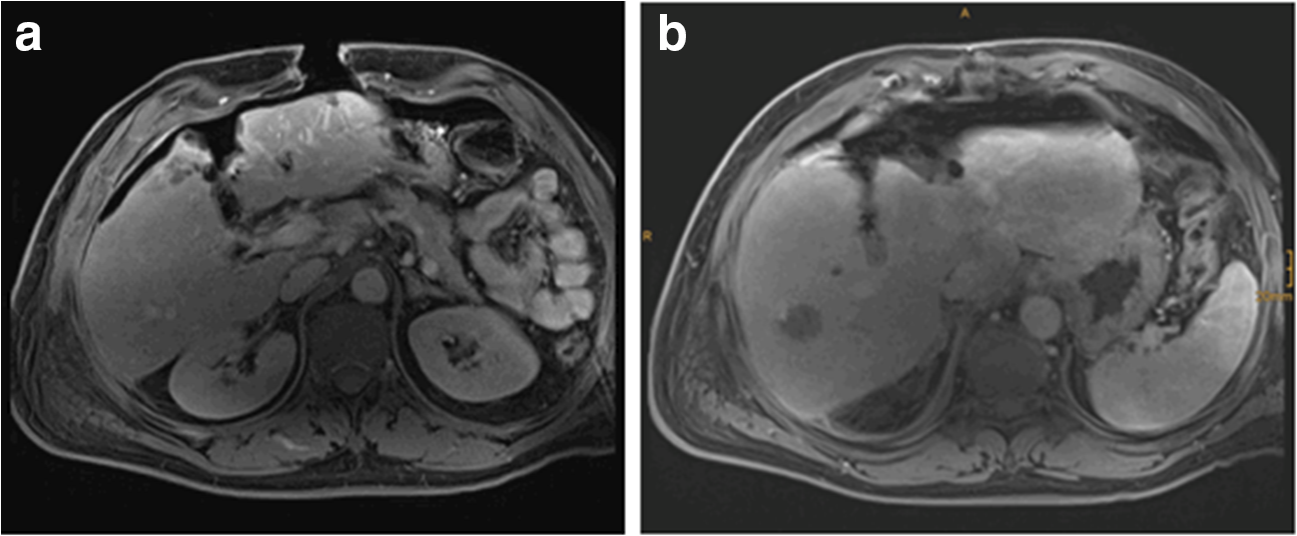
a–b ioMRI of the liver (19.09.2019) (a) and T1-weighted transversal plane after administration of 8 ml Gadovist i.v. (b)
